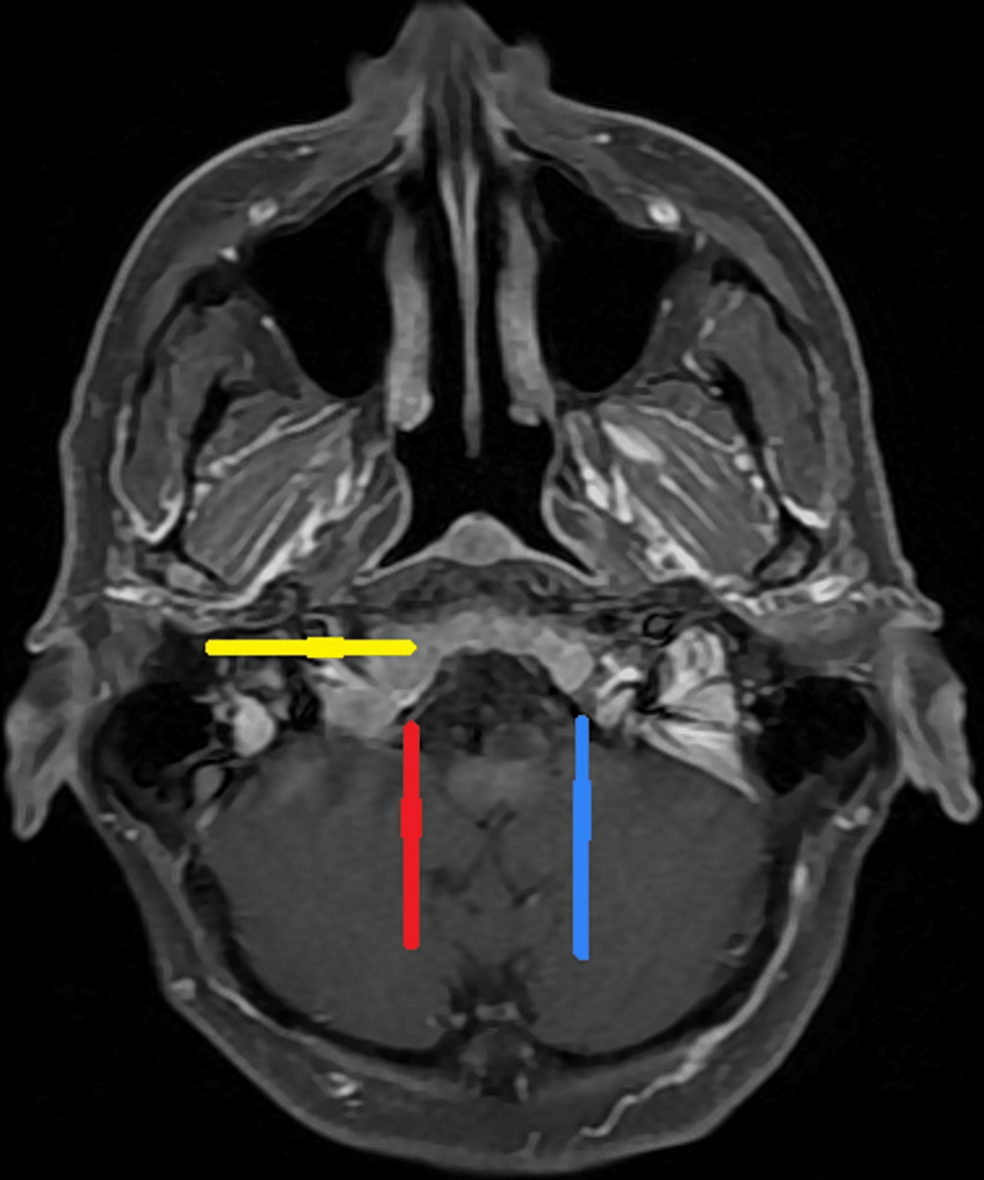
MRI revealing right hypoglossal canal narrowing due to diffuse bone marrow expansion. Axial MRI showing stenotic right hypoglossal canal (red arrow), the normal left hypoglossal canal (blue arrow), and diffusely abnormal bone marrow signal in clivus (yellow arrow)
